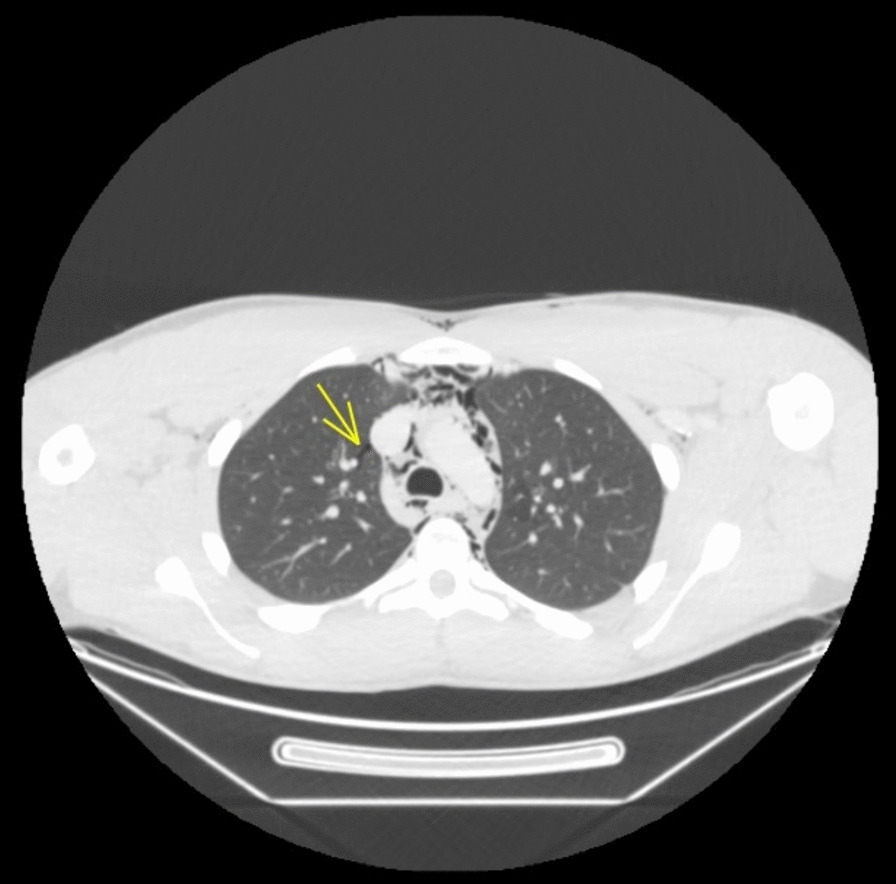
Computed tomography axial image of chest demonstrating pneumomediastinum and mild pneumopericardium. Yellow arrow: pneumomediastinum within the left superior mediastinum
